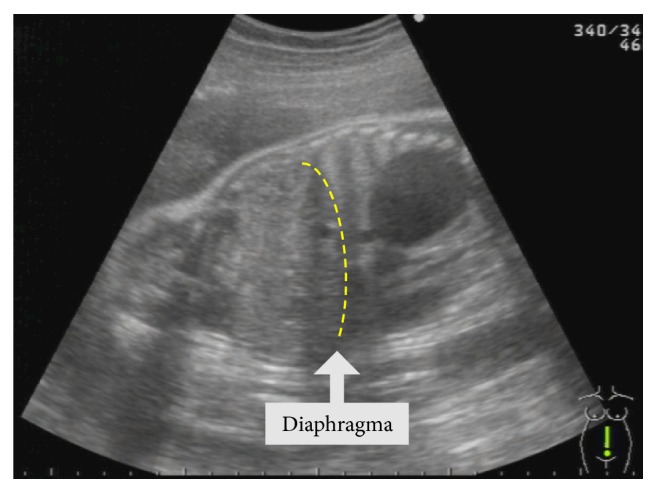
Ultrasound examination shows a 39 × 30 × 44-mm sized monocystic lesion in the mediastinum, in which the aortic arch was displaced upward.
